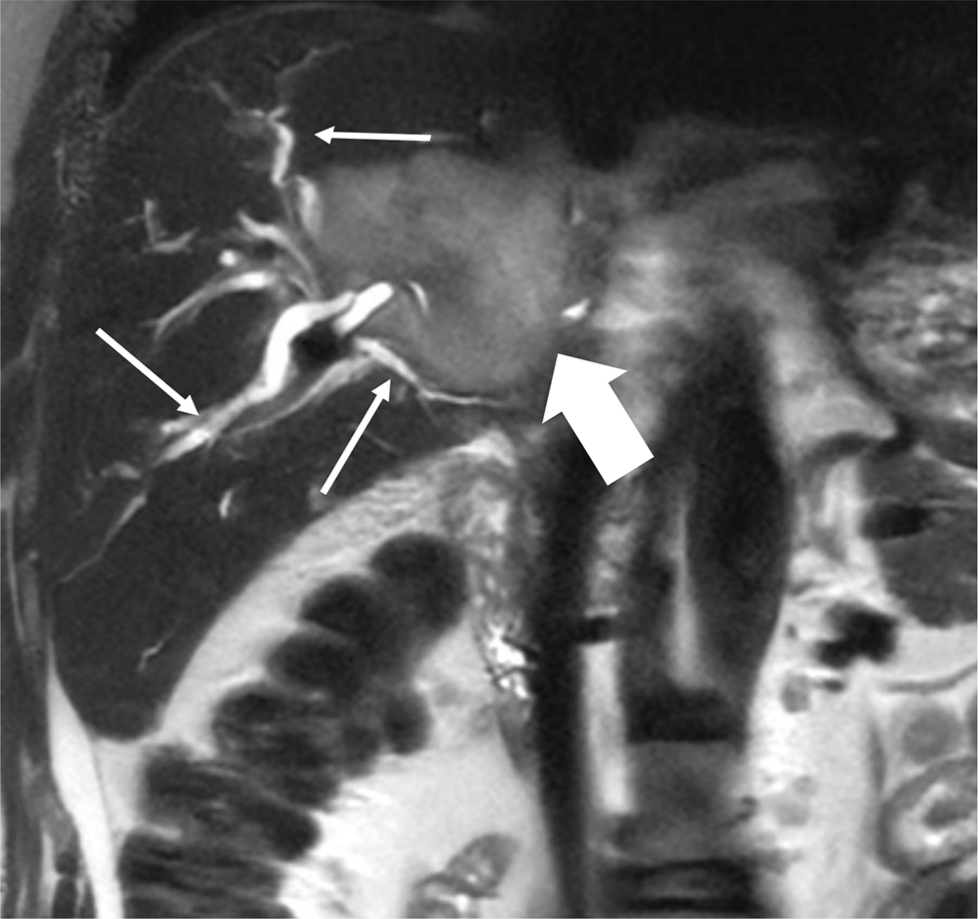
Dilated Upstream Bile Ducts. Coronal T2-weighted image demonstrates a large mildly T2 bright mass forming CCA in the right hepatic lobe (block arrow) causing dilated upstream bile ducts (arrows)
